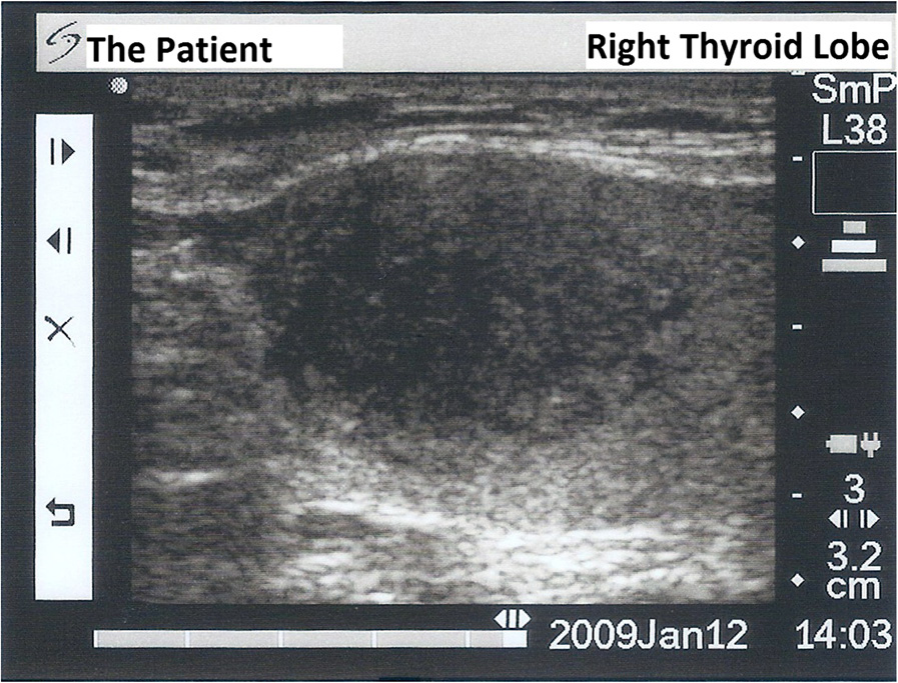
Thyroid ultrasonography image of right thyroid lobe
